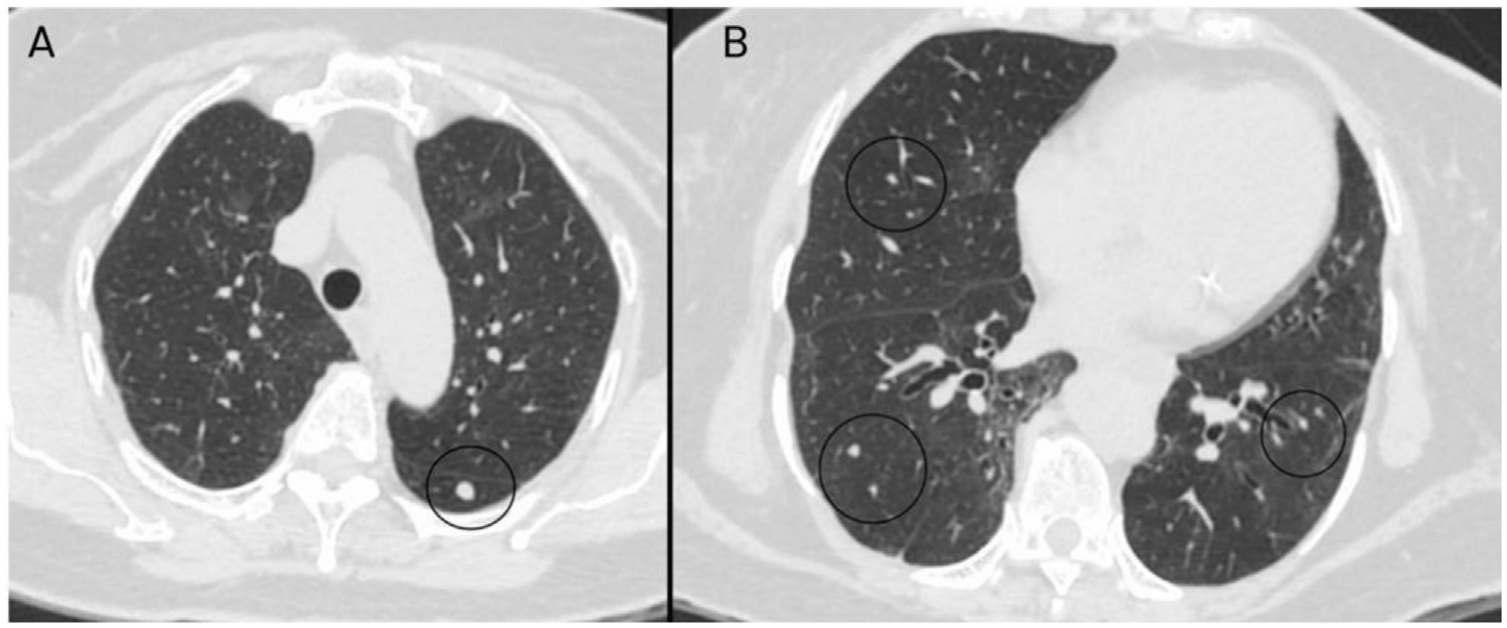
Chest CT scan performed for staging purposes. In lung window: multiple solid nodules (black circle) with well-defined margins are identified, distributed in isolation across both lung fields. These nodules measured between 5 and 9 mm. Additional findings include laminar atelectasis. A: Axial view at the apices. B: Axial view at the level of T9.
